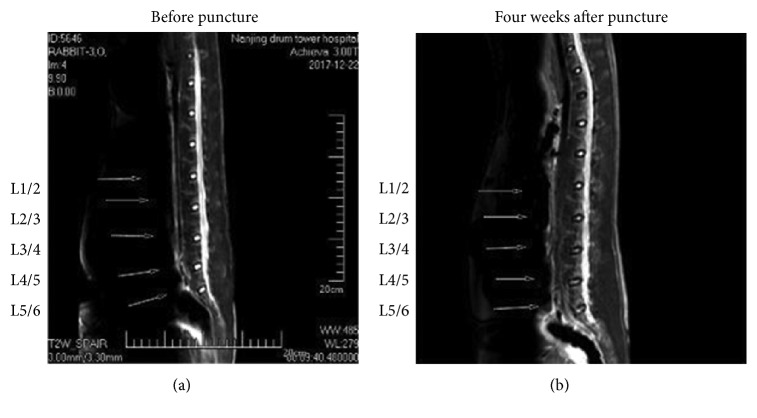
The signal of intervertebral discs (L2/3, L3/4, L4/5, and L5/6) in magnetic resonance (MR) T2-weighted images appeared to be significantly lower in (b) compared with (a), while in intervertebral discs L1/2, magnetic resonance images showed no difference on T2-weighted images.
